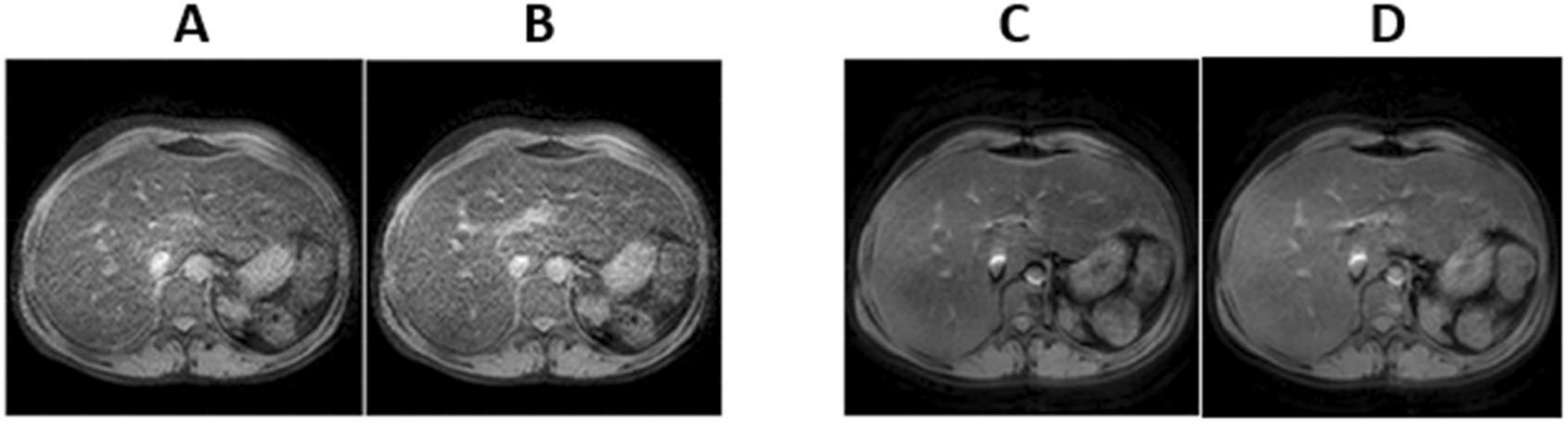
MRI images of the same patient using: (A) standard T2*, (B) optimised T2*, (C) standard T2 and (D) optimised T2 sequences.
